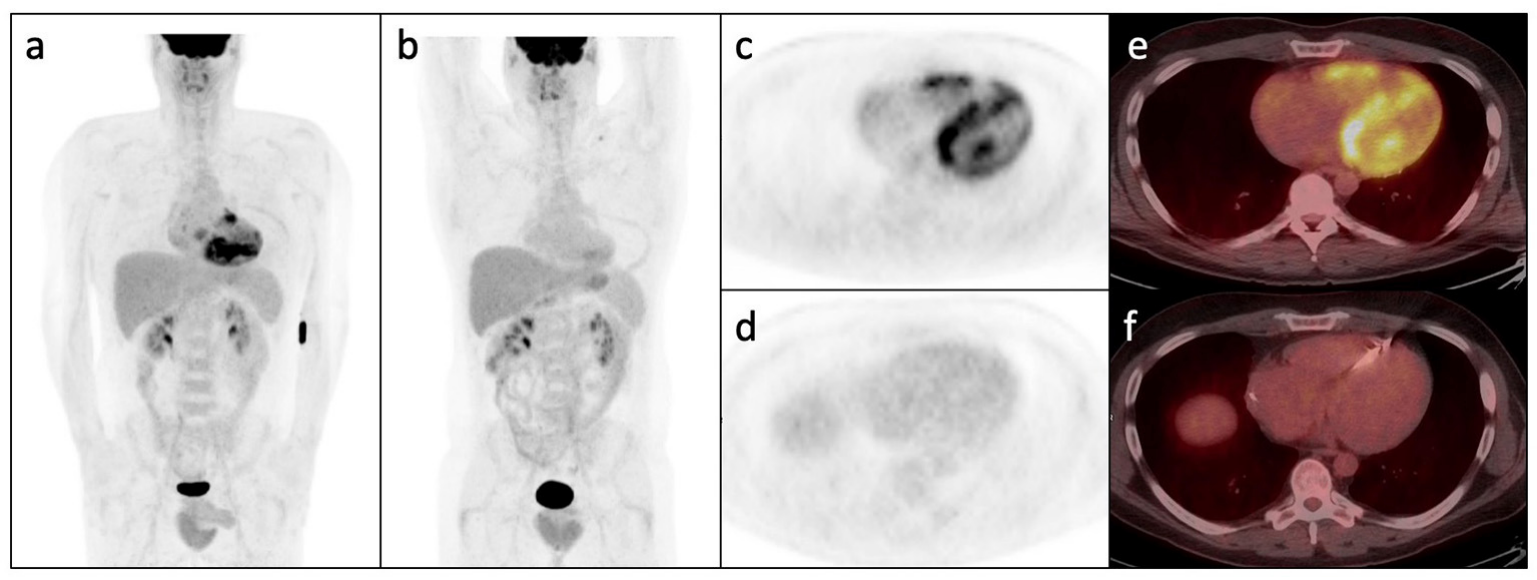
Whole-body FDG PET (a) and axial PET and PET/CT images (c,e) following appropriate pre-test preparation demonstrating abnormal patchy increase in FDG avidity within the right and left ventricular myocardium with subtle increase in right atrial FDG uptake. (b,d,f) Show post-treatment FDG PET/CT images in the same patient demonstrating interval resolution of the previously seen abnormal myocardial FDG uptake. Note interval ICD placement.
